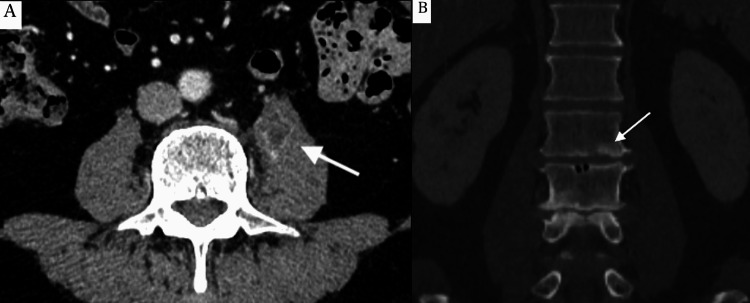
Computed tomography imaging findings (A) A ring-enhancing lesion in the left psoas muscle at the L2/3 level on contrast-enhanced computed tomography (arrow). (B) Deforming changes such as narrowing of the disc space and osteophyte formation were observed. Sclerosis and slight irregularities at the endplates of the L2/3 vertebral bodies were also observed (arrow).
